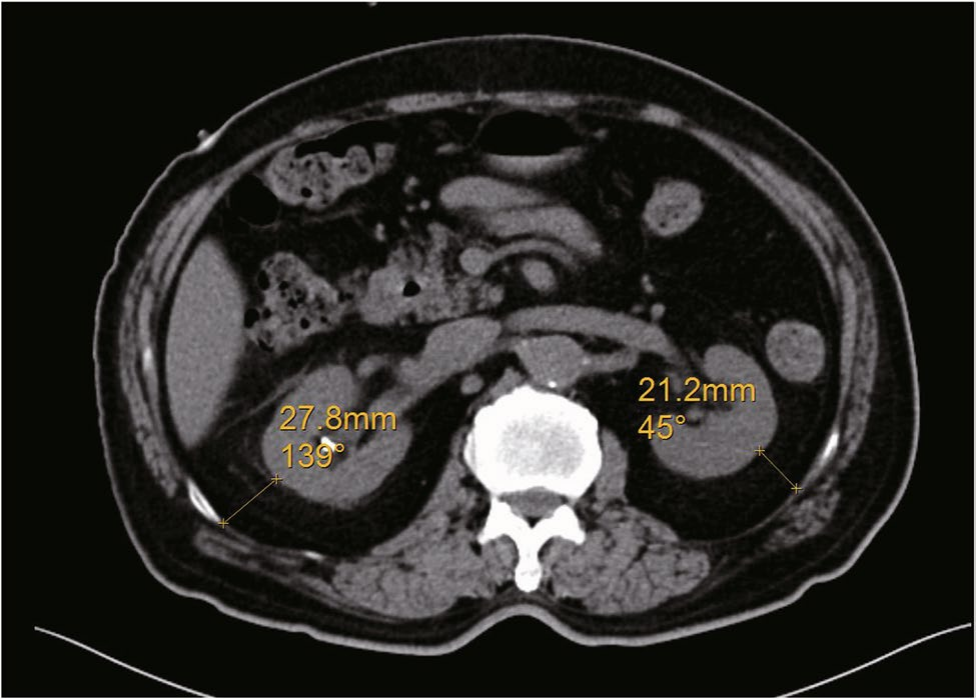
PFT at the renal hilum level can be evaluated by means of transverse CT imaging. The yellow line runs from the renal capsule to the sidewall. PFT: perinephric adipose tissue; CT: computed tomography
